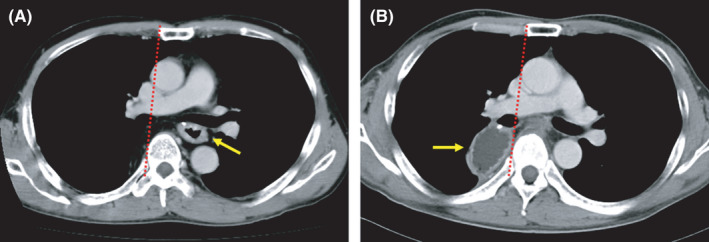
Computed tomography images of the gastric conduit. (A) DGC‐negative case. The gastric conduit (arrow) is in the usual posterior mediastinal space. (B) DGC‐positive case. The gastric conduit (arrow) is located outside of the line on the right edge of the sternum and thoracic vertebral body (dotted line) on the axial view. DGC, dislocation of the gastric conduit
